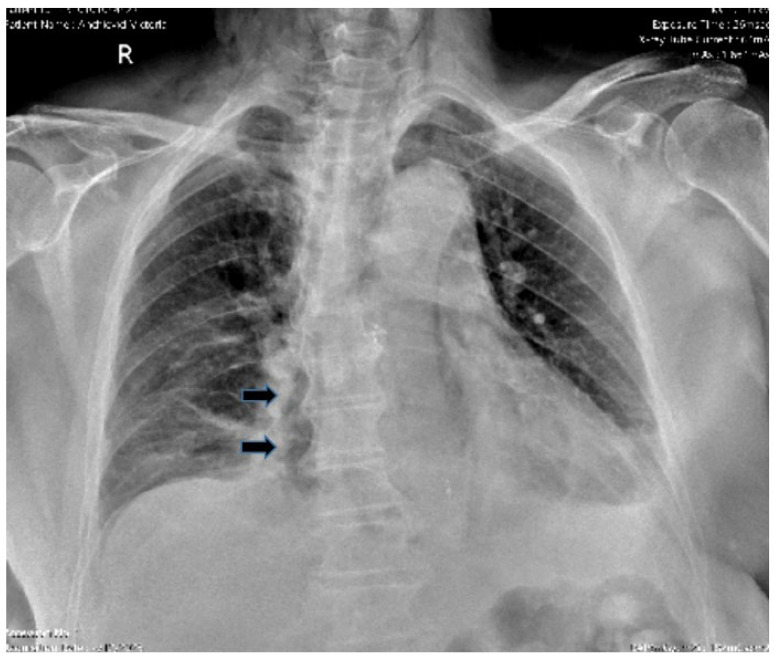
Chest X-ray. Mediastinal aerial image (arrows) is observed that “capes” the esophageal contour, suggestive of an esophageal perforation.
